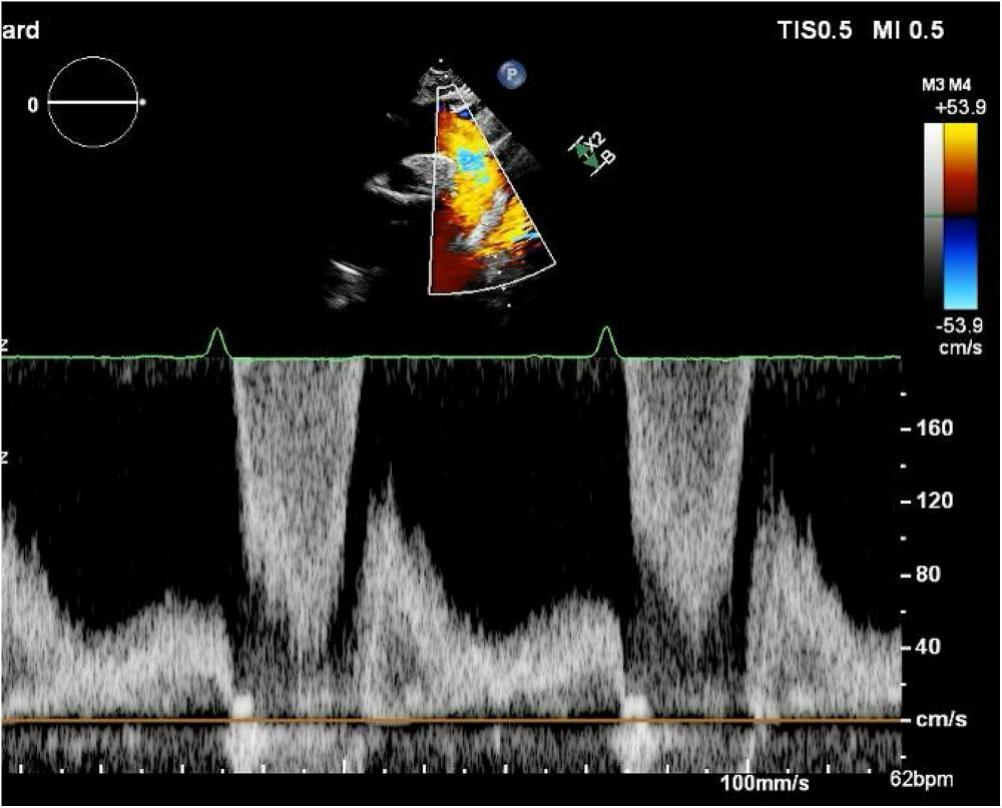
Doppler signal in aorta showing holodiastolic flow reversal (TTE, suprasternal view).
